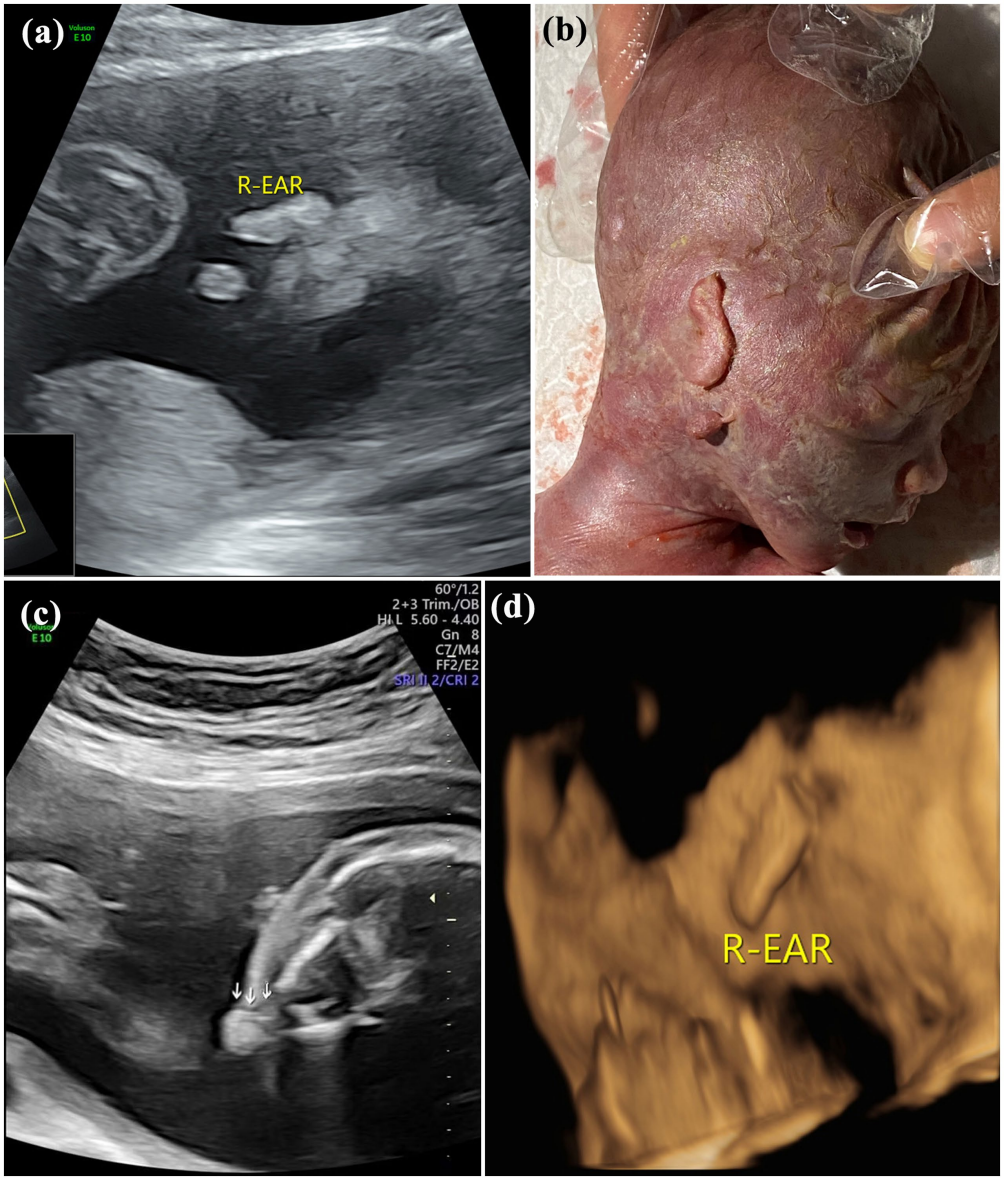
Images of type III of microtia: (A) 2D sonography and (B) photo (induced labor) of microtia type III accompanied by an accessory auricle; (C) 2D sonography of unilateral microtia type III accompanied by atresia of external acoustic meatus; and (D) 3D sonography of Unilateral microtia type III.
